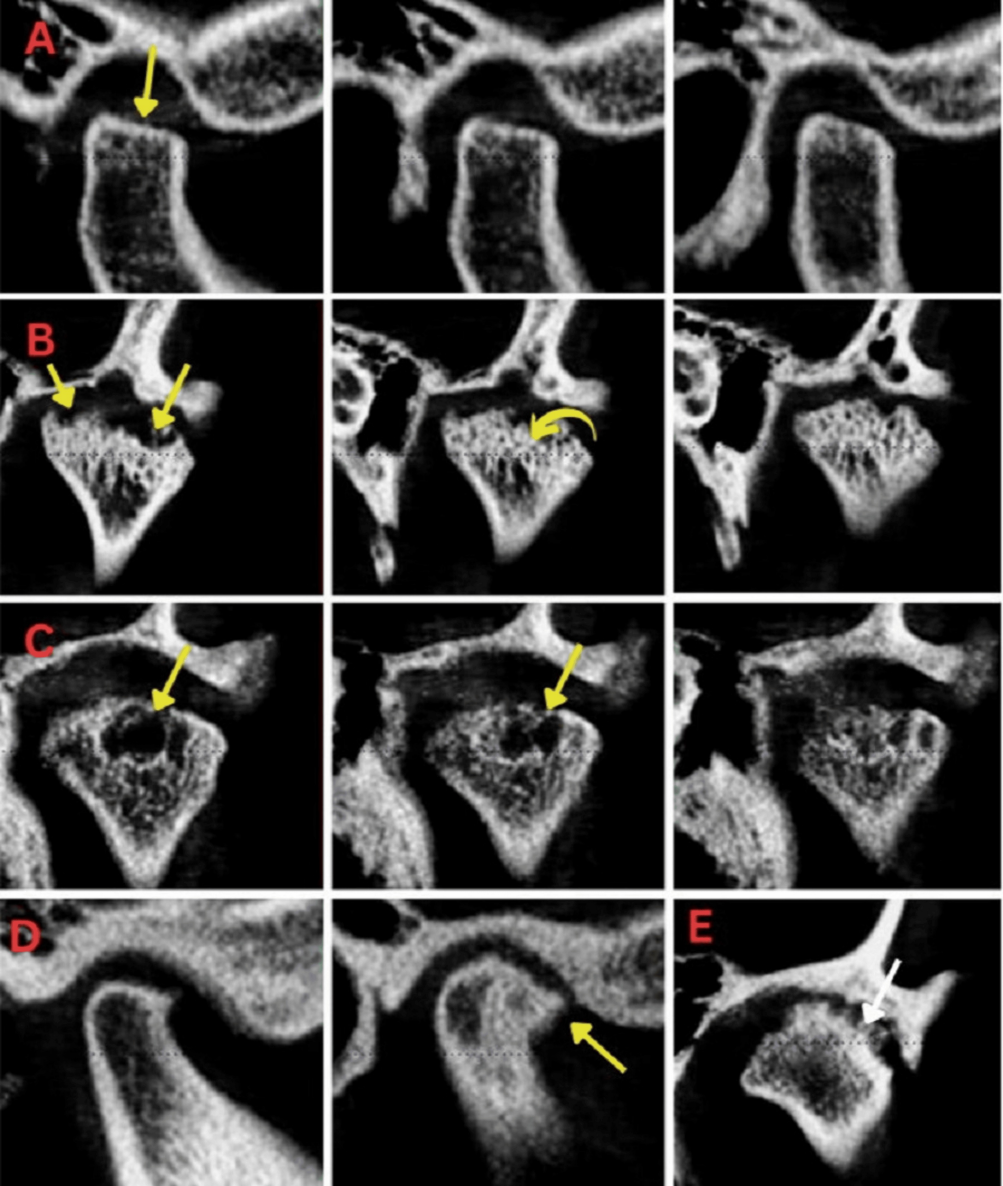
CBCT imaging showing TMJ Grade 3 A: Severe flattening of contours. B: Severe or multiple erosions (more than three). C: Large pseudocyst formation. D: Large osteophyte. E: Severe sclerosis of cortical outline. CBCT: cone-beam computed tomography; TMJ: temporomandibular joint.
